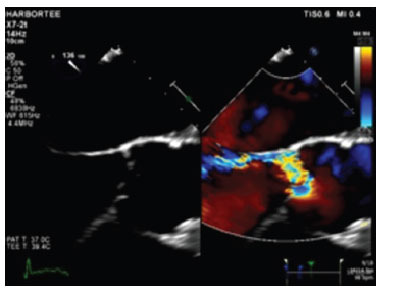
Transesophageal echocardiogram showing severe eccentric aortic regurgitation.
